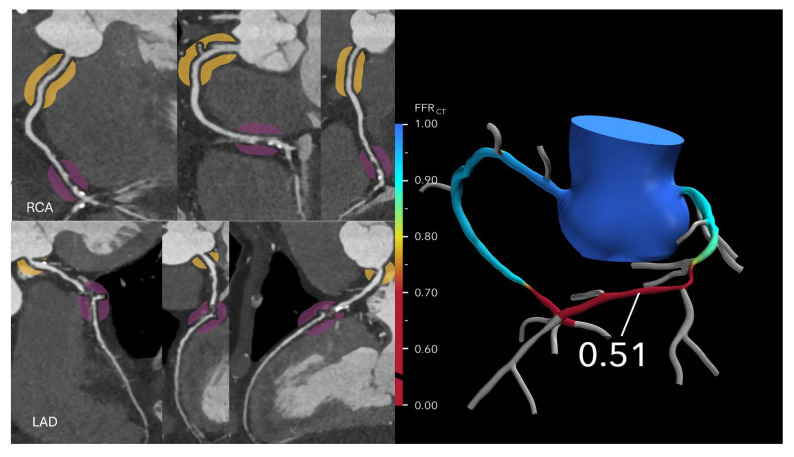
Optimizing treatment decisions with FFR-CT: (Left): A curved MPR coronary CTA image in a 64-year-old man with stable chest pain reveals severe stenoses in the mid left anterior descending (LAD) artery and distal right coronary artery. (Right): The corresponding FFRCT image shows a value of 0.51 measured 2 cm distal to the lesion, indicating severely reduced flow. Based on these findings, the patient proceeded to invasive coronary angiography followed by intervention.
